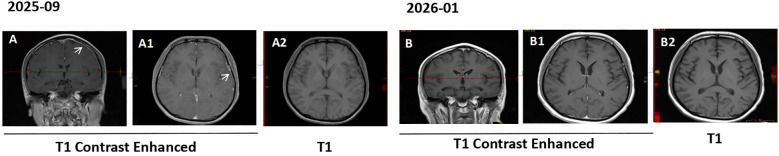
Post-contrast axial T1-weighted imaging (A, A1) ((A2) T1-weighted imaging) reveals abnormal leptomeningeal enhancement in the left cerebral hemisphere. Follow-up imaging performed 5 months later shows a normal brain appearance and reduced leptomeningeal enhancement in the left cerebral hemisphere on post-contrast axial T1-weighted imaging (B, B1) ((B2) T1-weighted imaging).
